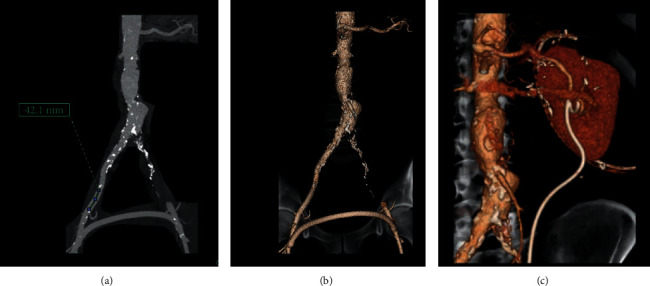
(a and b) represent the CT angiography (reconstruction) in a challenging case of a patient with a left external and common iliac artery chronic total occlusion who underwent femorofemoral bypass. In this case, transplantation in the intact right iliac artery segment (43.1 mm) could be associated with vascular steal following heterotopic transplantation. The image C corresponds to the post-OKT CT angiography.
